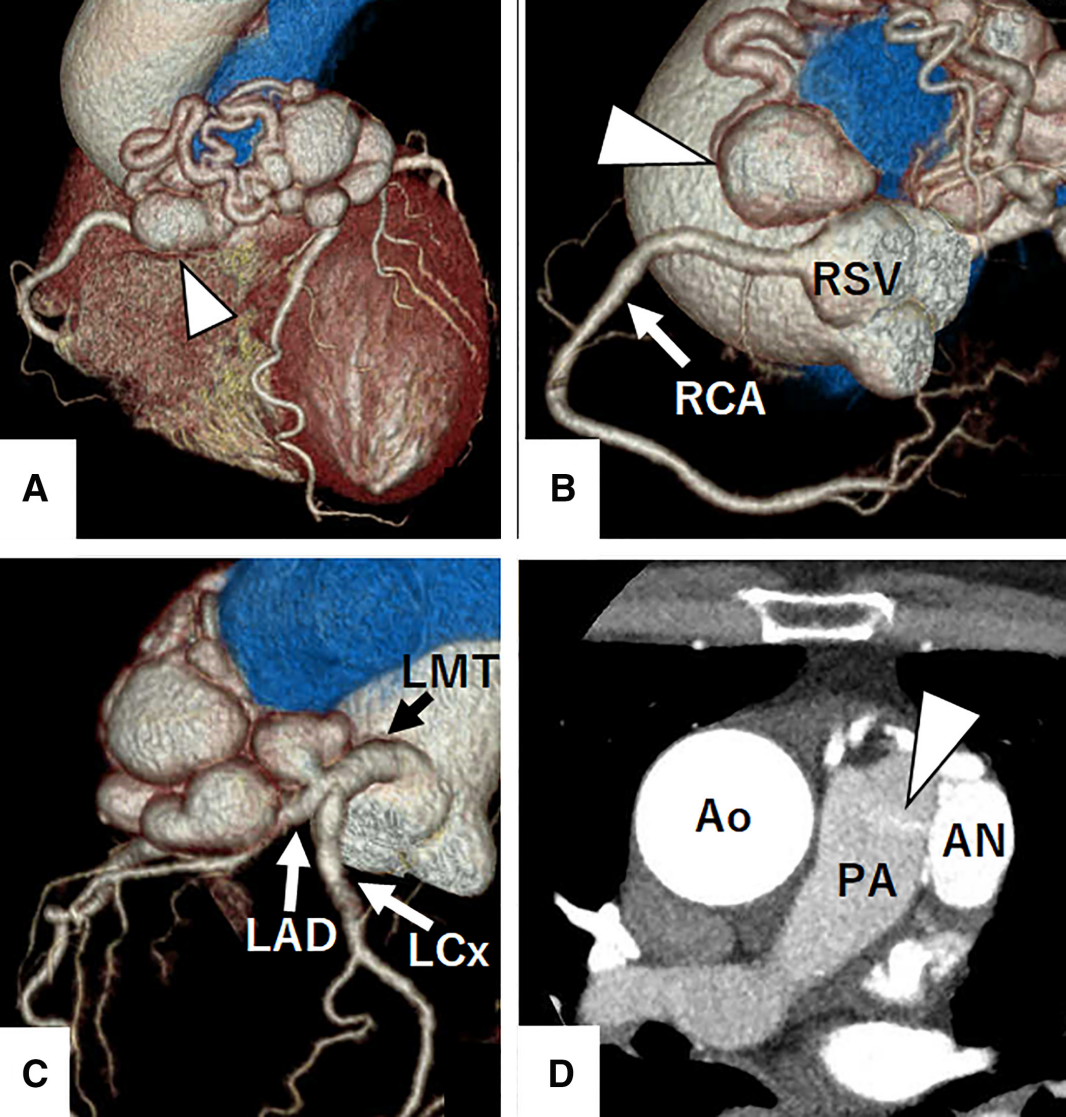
Preoperative contrast-enhanced CT findings. Contrast-enhanced CT reveals an aneurysm of the isolated conus artery, which arises directly from the right sinus of Valsalva (arrowheads in A, B). The vessel subsequently shows a beaded appearance with segmental dilatations and aneurysmal changes, and communicates with the proximal left anterior descending artery (C), forming Vieussens’ arterial ring. In the early phase of contrast enhancement, opacification of the main pulmonary artery is observed, indicating the presence of a coronary-to-pulmonary artery fistula (arrowhead) (D). AN, aneurysm; Ao, aorta; LAD, left anterior descending artery; LCx, left circumflex artery; LMT, left main trunk; PA, pulmonary artery; RCA, right coronary artery; RSV, right sinus of Valsalva
